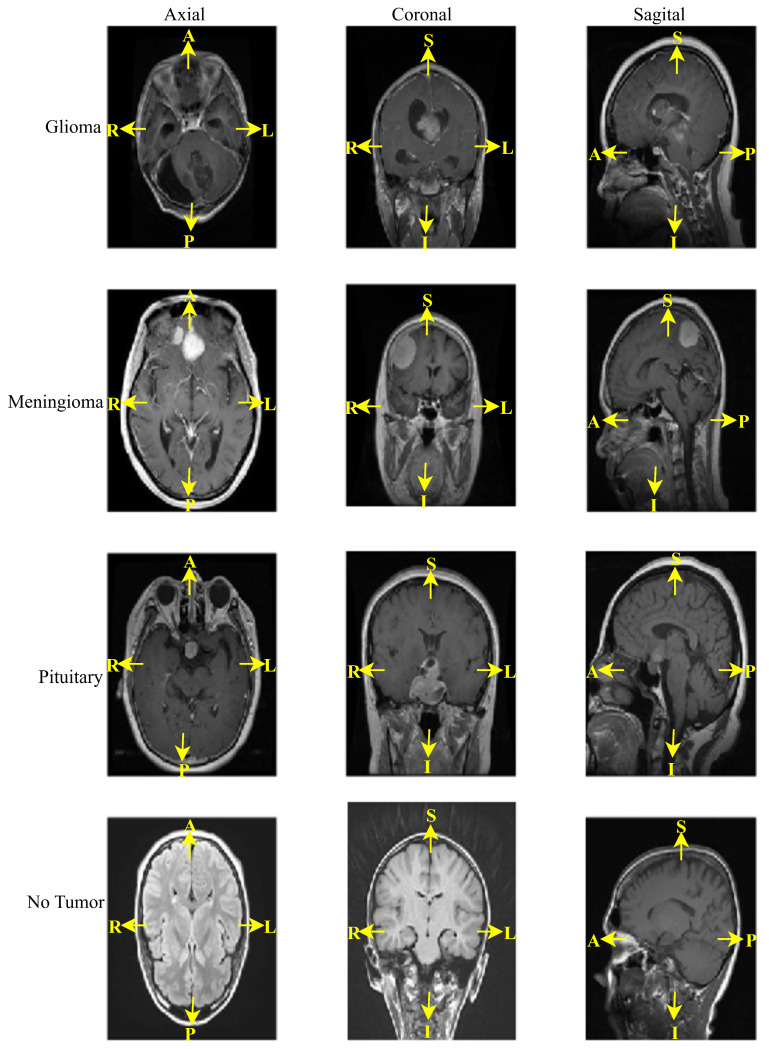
Representative MRI scans from the Kaggle multiclass brain tumor dataset across axial, coronal, and sagittal views. Yellow arrows indicate anatomical orientation directions—A (anterior), P (posterior), R (right), L (left), S (superior), and I (inferior)—ensuring spatial interpretability of brain regions.
